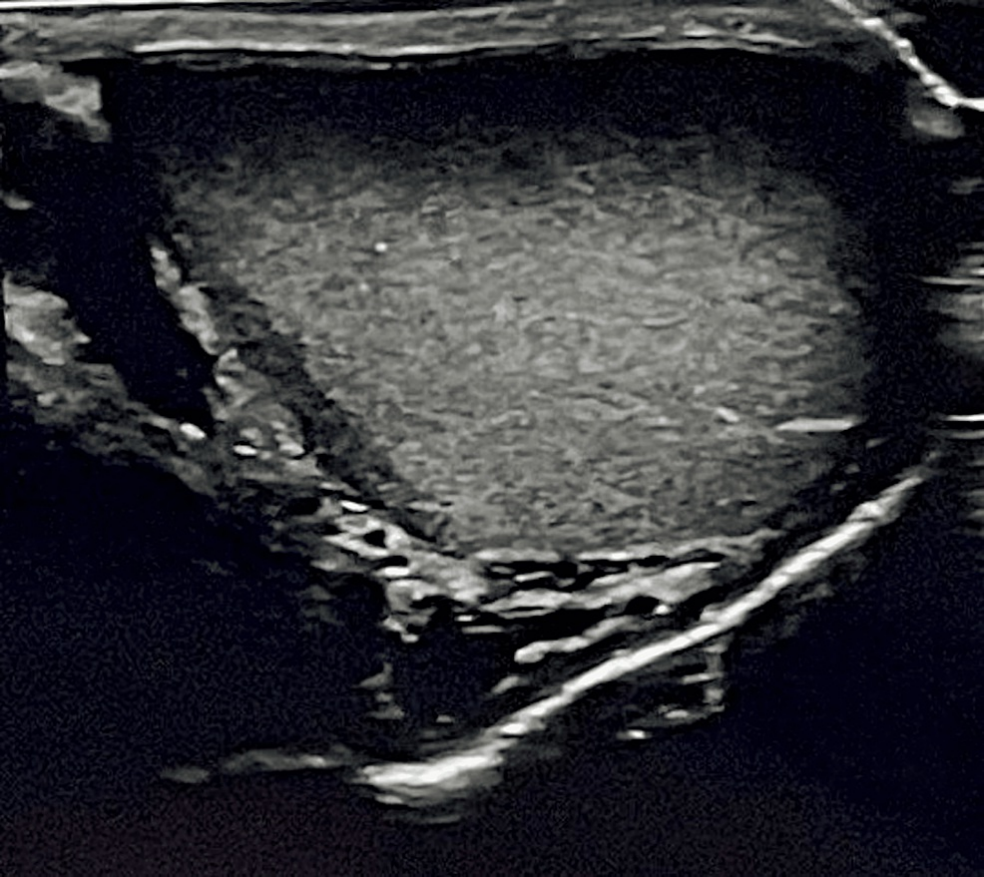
Ultrasonographic image of the left testicle.
